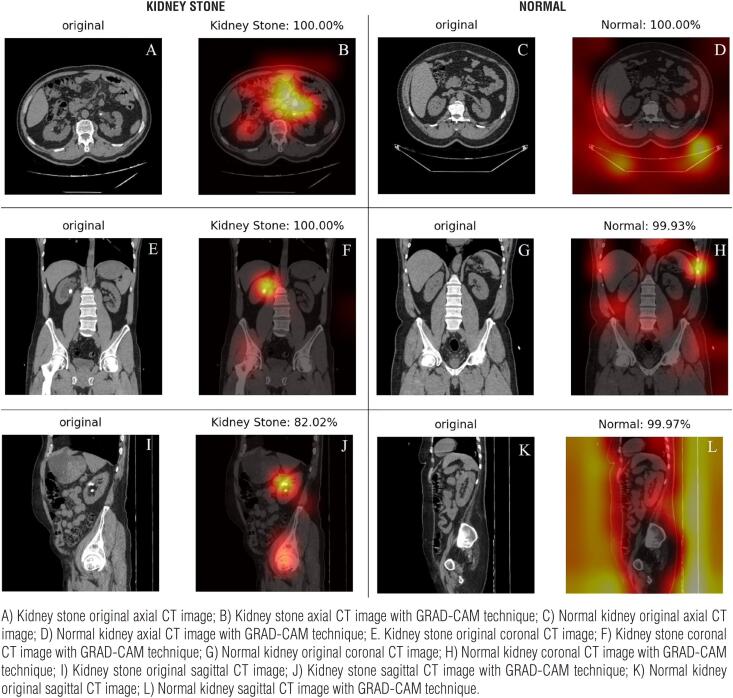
Axial, sagittal, and coronal CT images of the patients bearing 1–2 cm-sized kidney stones and without kidney stones, demonstrated with the Grad-CAM technique. Percentages refer to the estimates of the AI model.
